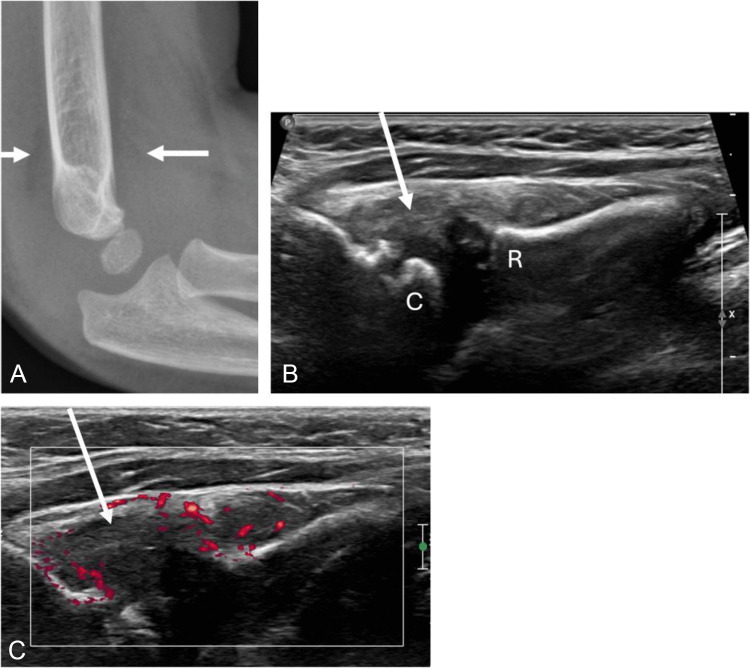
A 2-year-old girl with elbow swelling later diagnosed with oligoarticular juvenile idiopathic arthritis. A Lateral radiograph of the elbow shows elevation of the anterior and posterior fat pads (*arrows*), consistent with joint effusion. B Long-axis ultrasound of the anterior elbow at the level of the radiocapitellar articulation (*R*, radius; *C*, capitellum) demonstrates a hypoechoic region of synovitis (*arrow*) within the anterior joint recess, with associated hyperemia evident on Doppler imaging (C)
